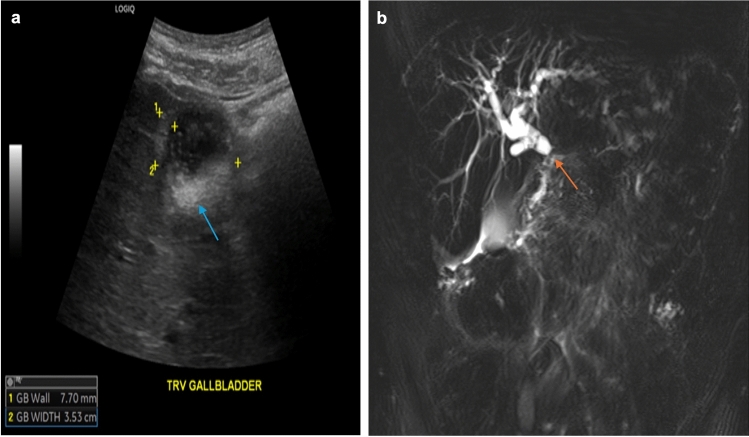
Findings related to fluoropyrimidine and platinum-based chemotherapy toxicity. Ultrasound (a) shows gallbladder sludge/stones (blue arrow) and wall thickening (calipers denote wall). MRCP (b) shows diffuse intrahepatic biliary ductal dilatation with stricture (orange arrow) in the periportal region
